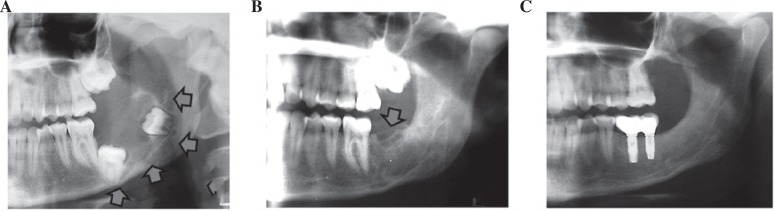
(A) Panoramic radiograph showing a multicystic ameloblastoma in the left mandibular angle associated with the 2nd and 3rd impacted molar (arrows). (B). Recurrent multicystic ameloblastoma located in the mandibular ridge (arrows). (C) Radiographic control after marginal mandibulectomy and reconstruction with cancellous bone graft obtained from proximal tibia, placement of two dental implants and restoration with implant-supported prostheses.
